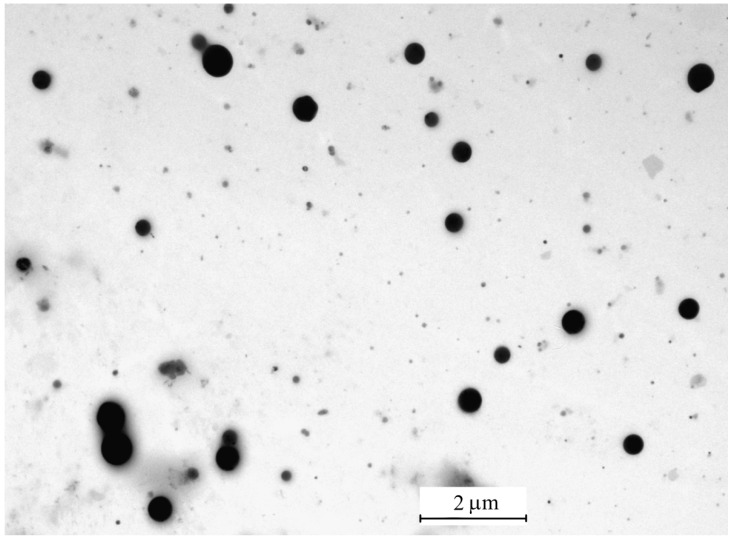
TEM image of dried aerosol particles formed by ultrasonic nebulizer.
